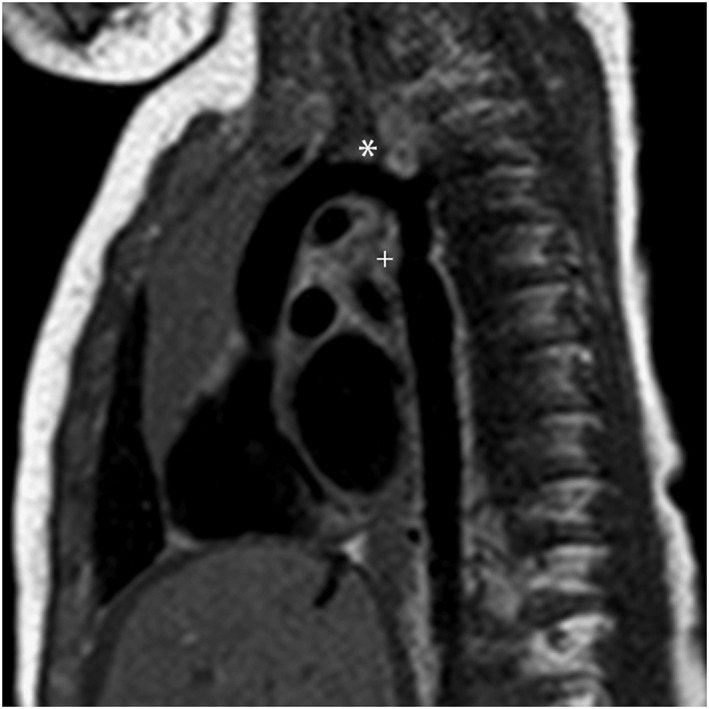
Postnatal MRI of the same patient at 5 months of age. No postnatal interventions have been performed. Despite prenatal findings, there is only mild coarctation, with isthmal hypoplasia and a posterior shelf at the point of insertion of the ductal ligament (+) (a vestige of the fetal arterial duct). The proximal aortic arch is within normal limits (*)
